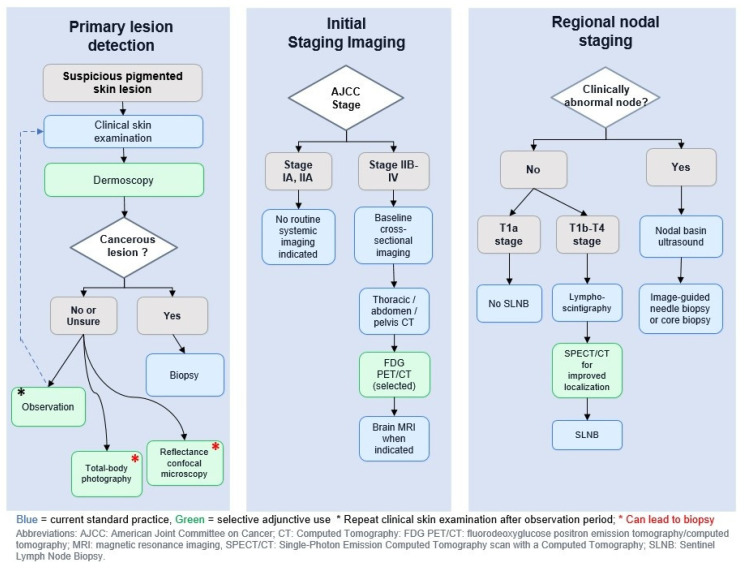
Clinical imaging pathway in cutaneous melanoma for primary lesion detection, initial staging, and regional nodal staging. Blue boxes represent current standard practice, green boxes represent selective adjunctive, * (black) shows that clinical skin examination is repeated after the observation period and * (red) shows that the TBP and RCM results can lead to biopsy. Note: AJCC: American Joint Committee on Cancer; CT: computed tomography; FDG PET/CT: fluorodeoxyglucose positron emission tomography/computed tomography; MRI: magnetic resonance imaging, SPECT/CT: single-photon emission computed tomography scan with computed tomography; SLNB: sentinel lymph node biopsy.
